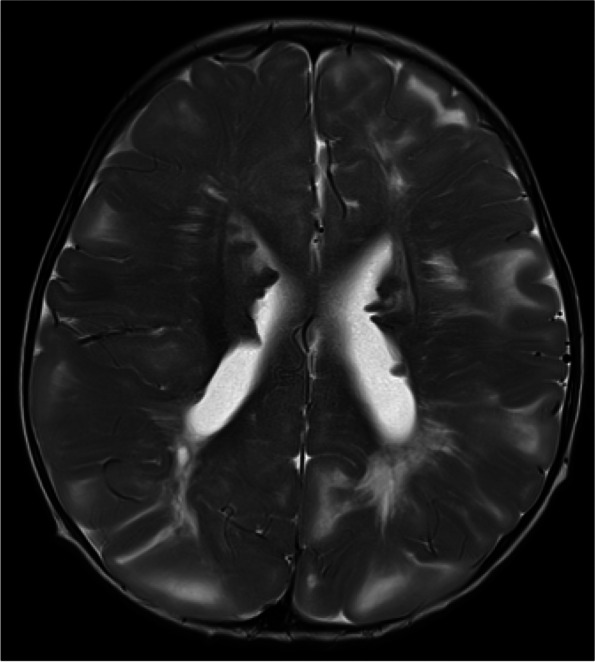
MRI brain axial T2 sequence demonstrates the typical findings of tuberous sclerosis (TS), including subcortical T2 high signal tubers and subependymal nodules lining the lateral ventricles
